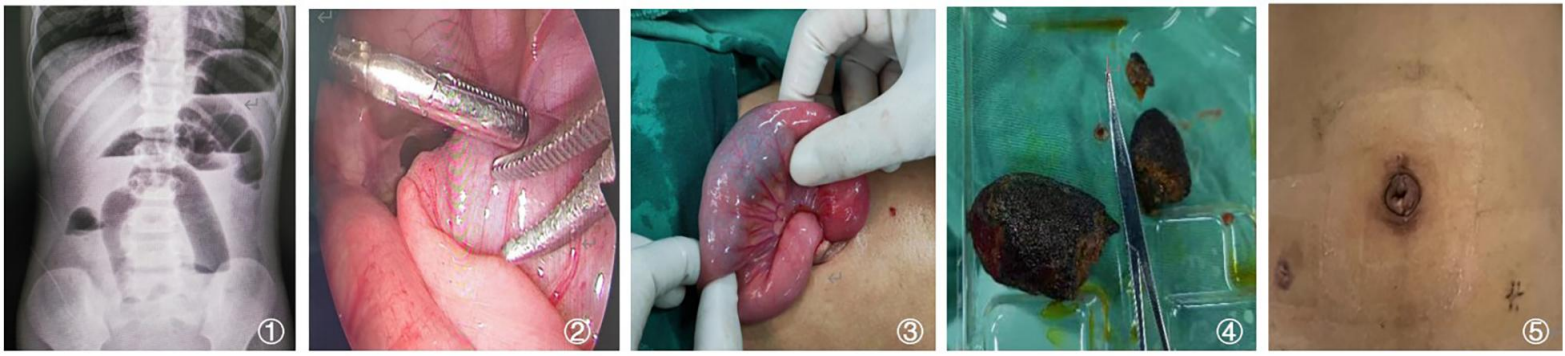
(1) Abdominal X-ray showing complete intestinal obstruction. (2) Intraoperative view showing the transition zone between dilated and non-dilated small bowel. (3) Exteriorization of the affected bowel segment containing the gastrointestinal foreign body through the umbilical incision. (4) Identification of foreign body as tannic acid fecalith. (5) Appearance of postoperative abdominal wound at 1 week. SBO, small bowel obstruction. A few panels were adapted from Dong et al. (13), under CC BY 4.0.
